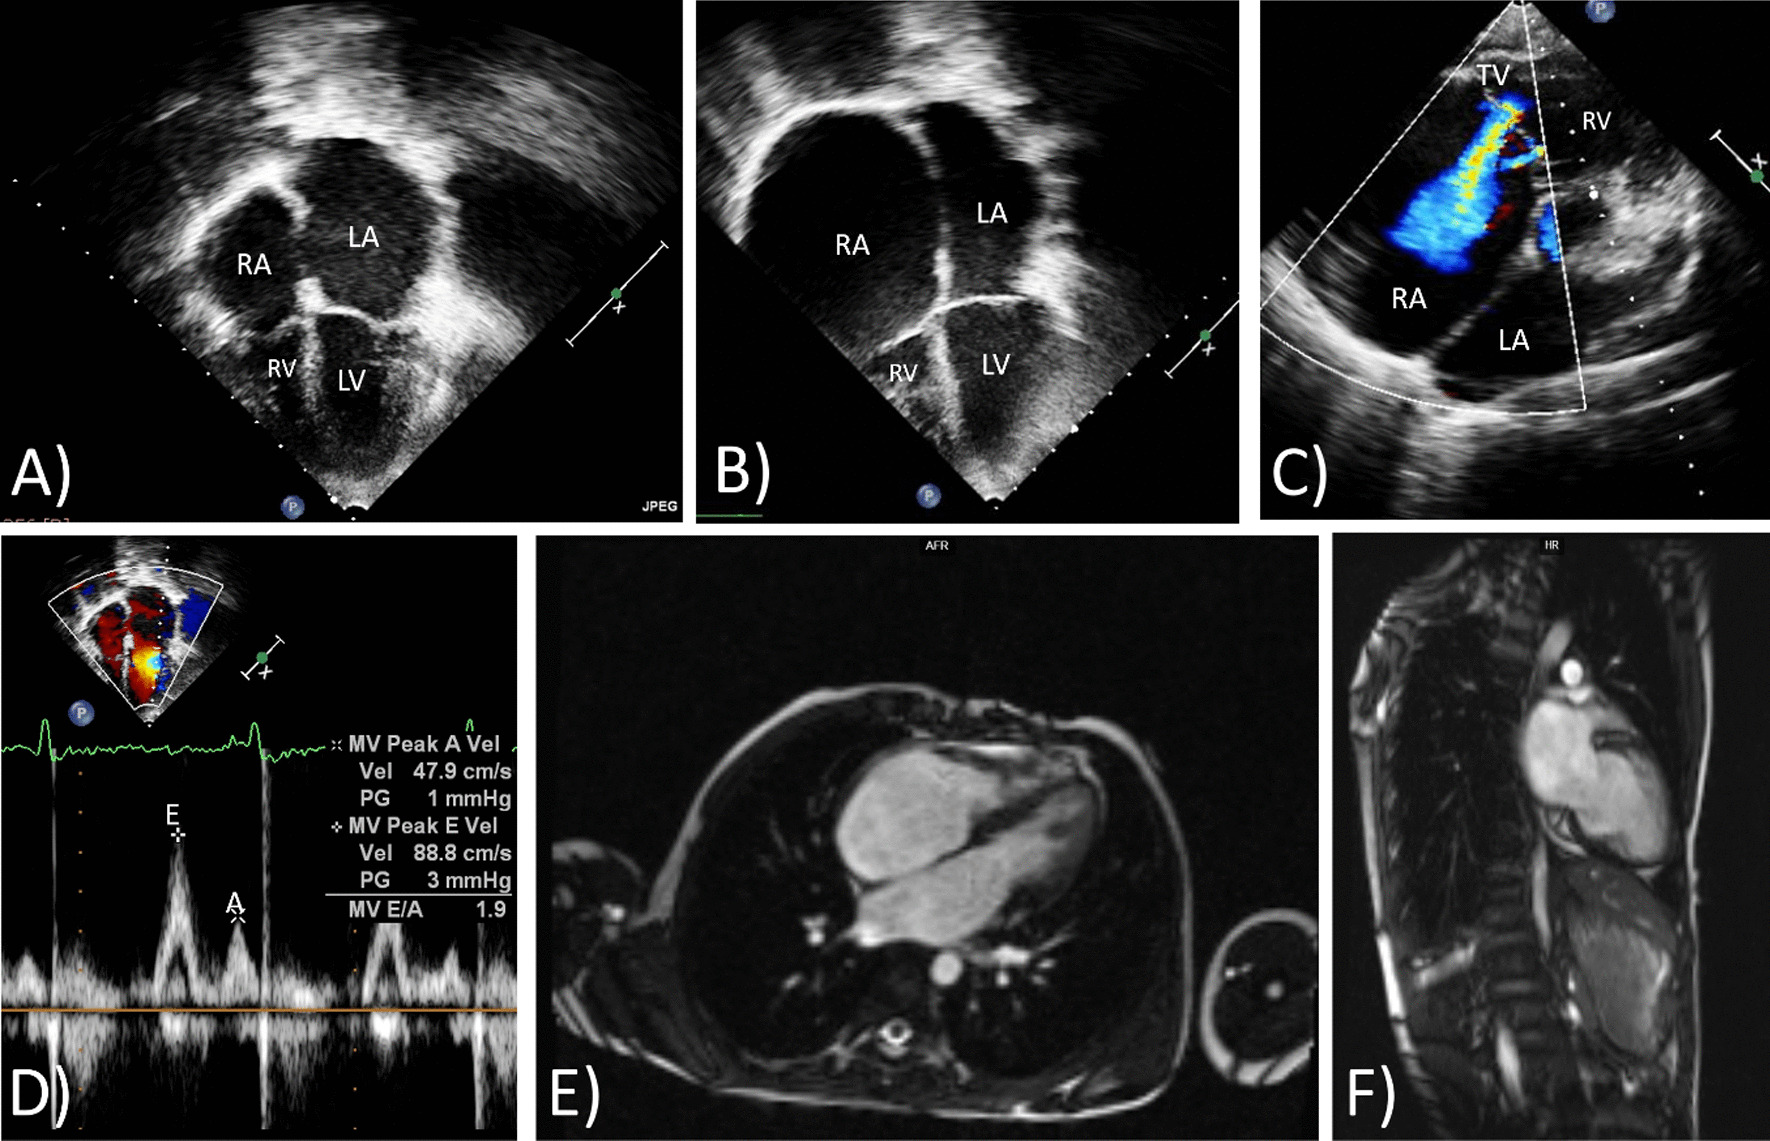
Cardiac imaging features of restrictive cardiomyopathy in Patient 7 (b, c, e, f) and Patient 10 (a, d). Images of patient 10 corresponding to four-chamber view (a) and Pulsed Way Doppler on mitral valve (d) confirmed atrial enlargement and moderate left ventricle diastolic dysfunction. Echocardiography pictures of patient 7 (b, c) corresponding to four-chamber view (b) and short-axis view with Color Doppler (c) also confirmed atrial enlargement with normal ventricle wall thickness and severe tricuspid valve regurgitation. Cardiac MRI images of patient 7 (e, f) demonstrating atrial enlargement and normal ventricle wall thickness. *RA* right atrium, *LA* left atrium, *RV* right ventricle, *LV* left ventricle, *TV* tricuspid valve
